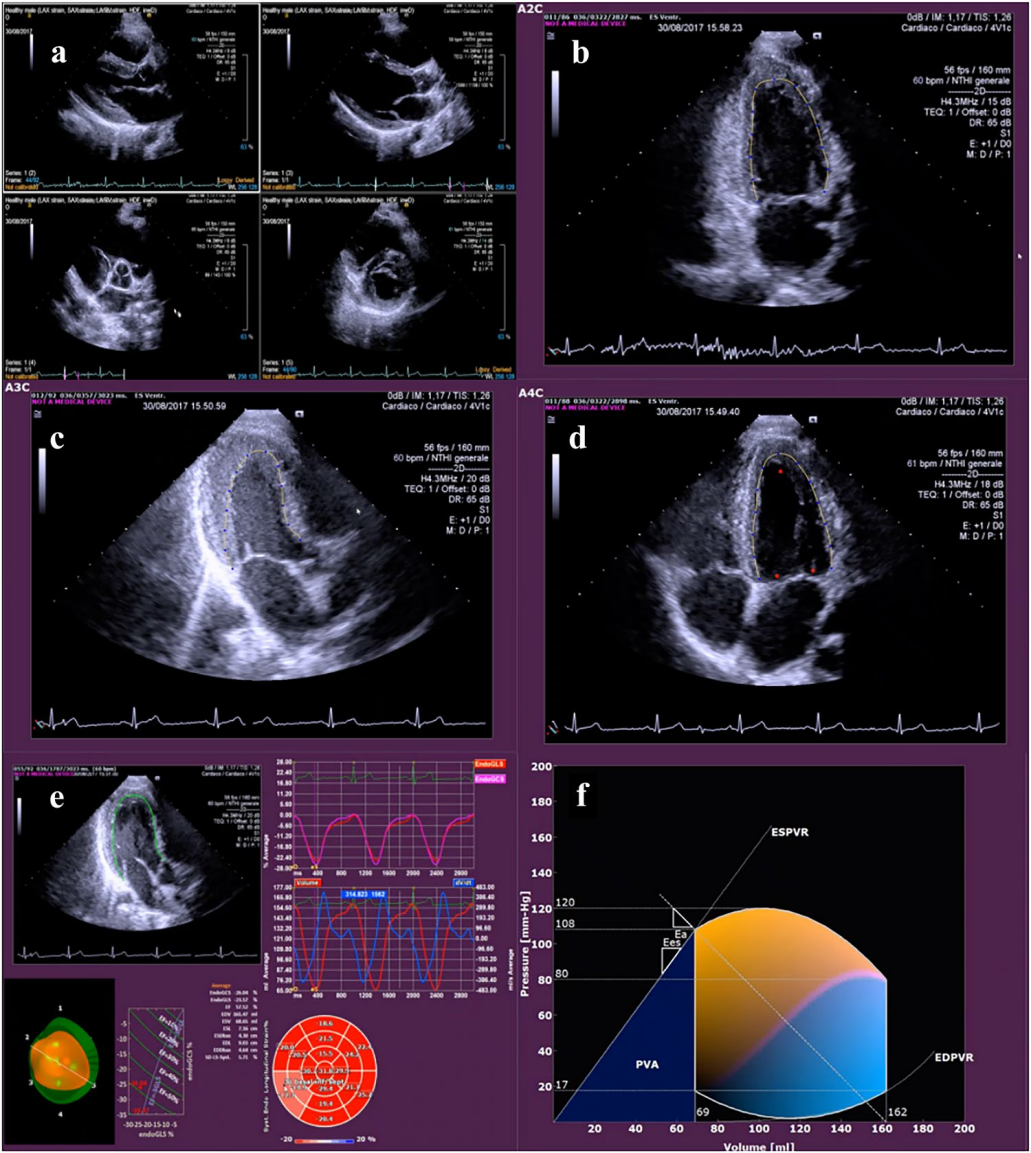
The steps from the echocardiographic images acquisition to the *P/V* loop reconstruction. The process includes: a acquisition of 2D echocardiographic images (apical 4-chamber, 2-chamber and long-axis); b–d analysis of the echocardiographic images by endocardial border delineation and speckle tracking (QStrain, Medis BV, Leiden, NL); e display of strain parameters; f reconstruction of the *P/V* loop using the single-beat algorithms [66]
